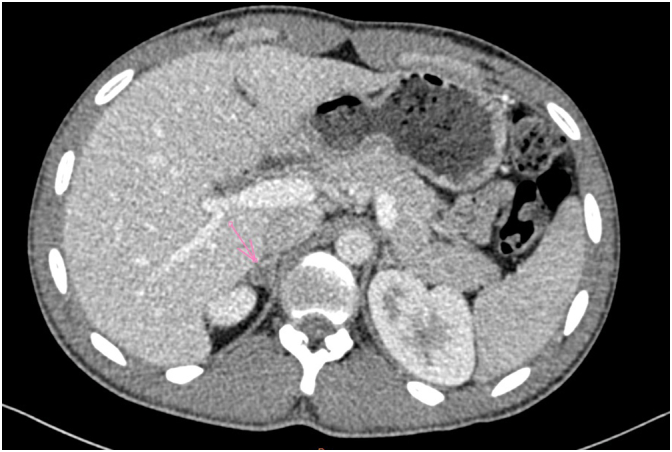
Axial view of abdominal CT scan was performed and showed a right adrenal cortical adenoma.
